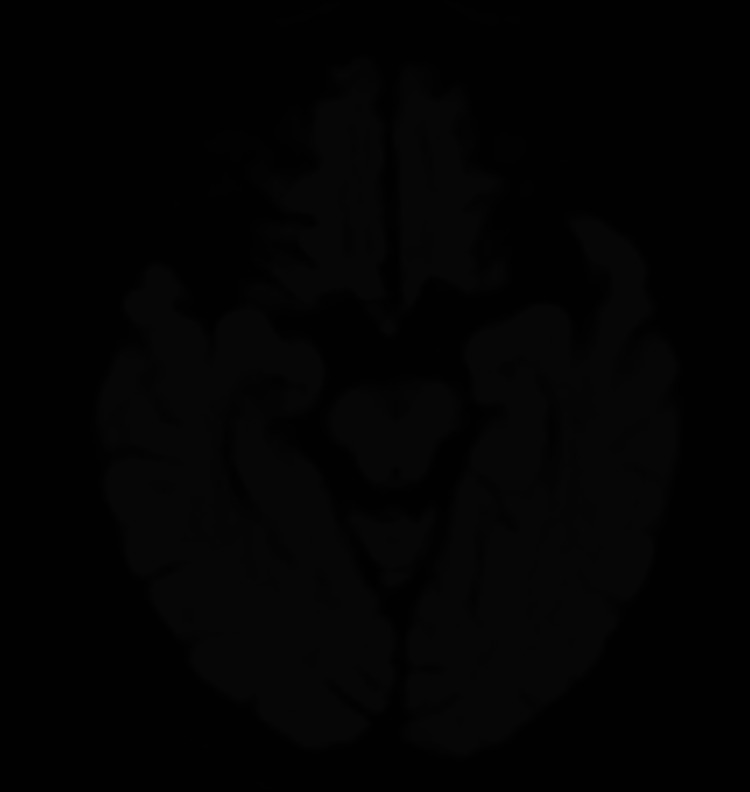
Negative MRI diffusion-weighted imaging (DWI) sequence at the level of mesial temporal lobes. No hippocampal abnormality noted. Study done 18 hours after symptom onset.
